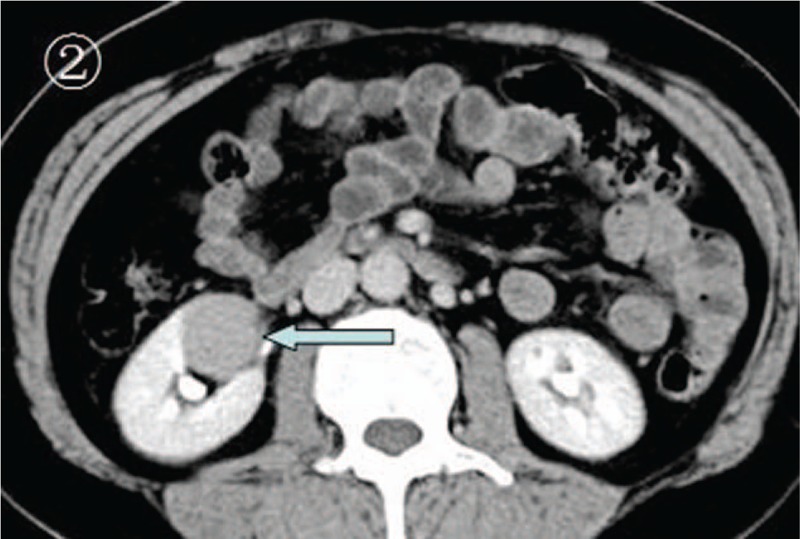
The abdominal postcontrast enhanced CT scan. This scan revealed no obvious enhancement of the mass, but showed a clear demarcation of the tumor from its surrounding normal tissues and a regular shape of the tumor (arrow). CT = computed tomography.
